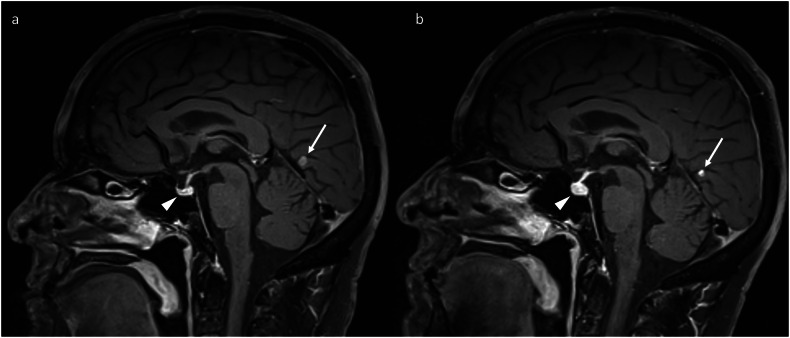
Sagittal post-contrast T1-weighted images before (a) and after (b) two cycles of combination ipilimumab and nivolumab for metastatic melanoma demonstrate enlargement of the pituitary gland (arrowheads) typical of hypophysitis, together with a decrease in the size of the occipital metastasis (arrows)
